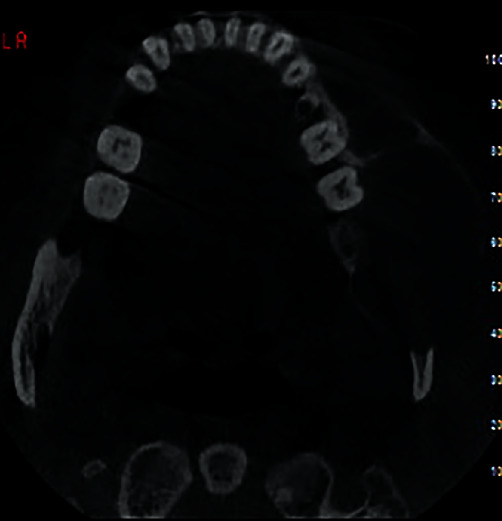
Axial CBCT section upon presentation.
